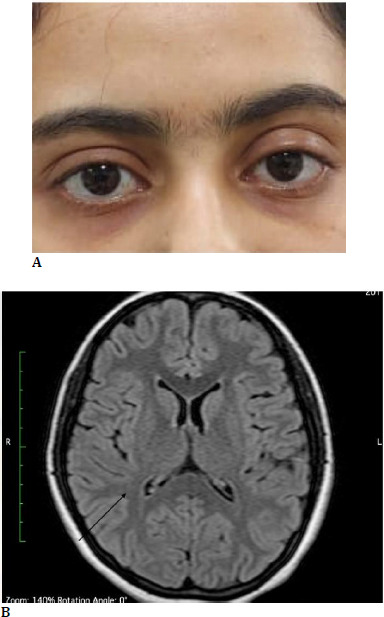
A. Bilateral mild ptosis with restriction of ocular movements in a young female; B. Axial FLAIR image - no abnormality is observed
